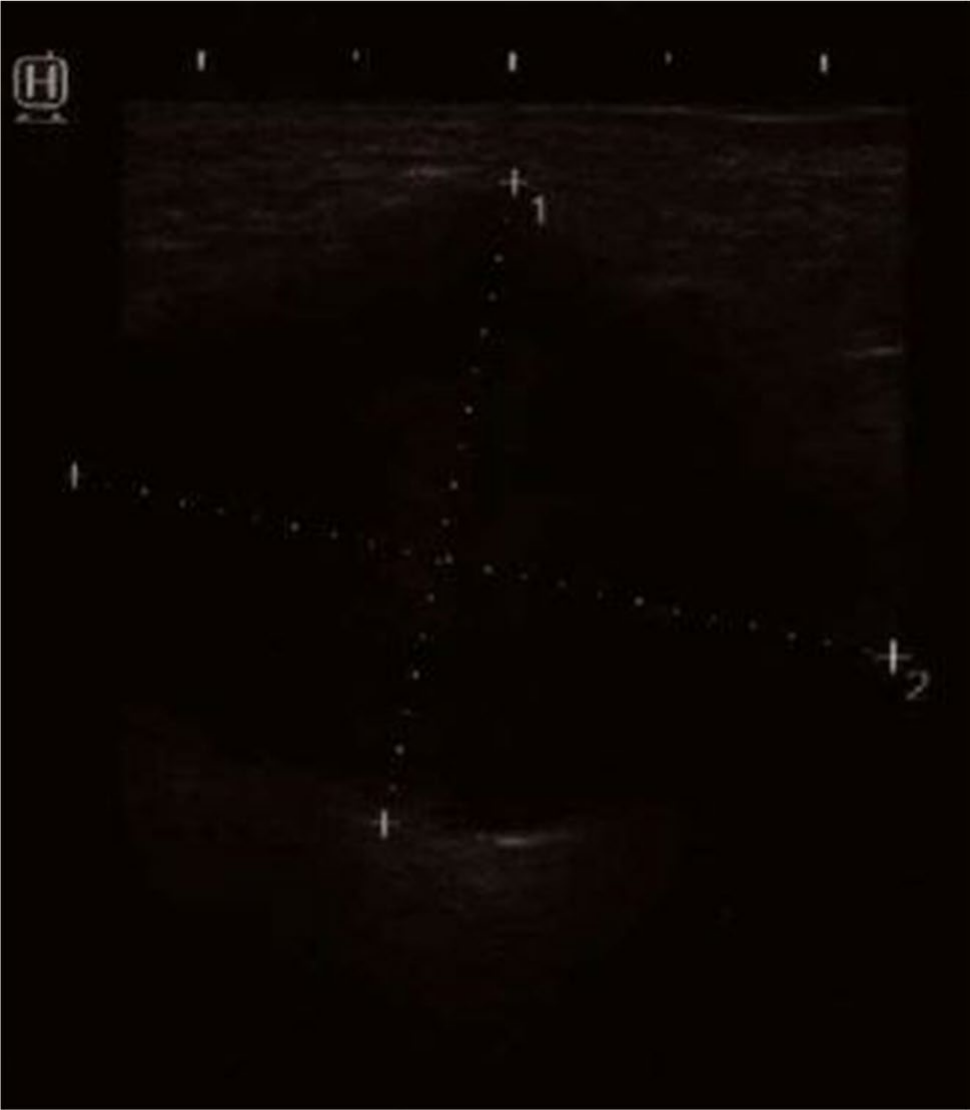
Sonographic image of the hypoechoic, solid mass of the left Bartholin gland
